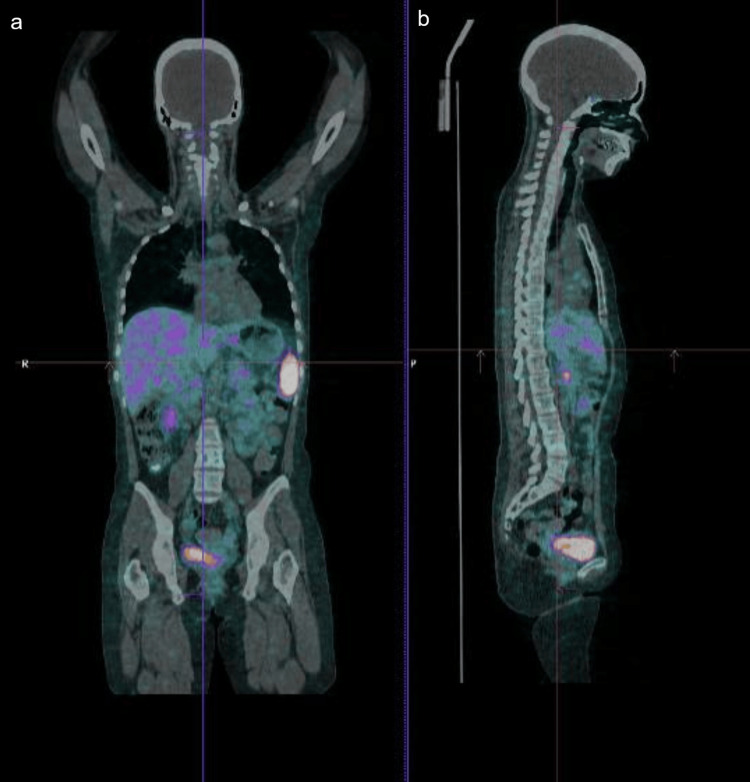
68Ga-DOTANOC PET/CT with coronal (a) and sagittal (b) views showing no evidence of lesions with increased somatostatin receptor expression. ^68^Ga-DOTANOC PET/CT: gallium-68 DOTANOC positron emission tomography.
